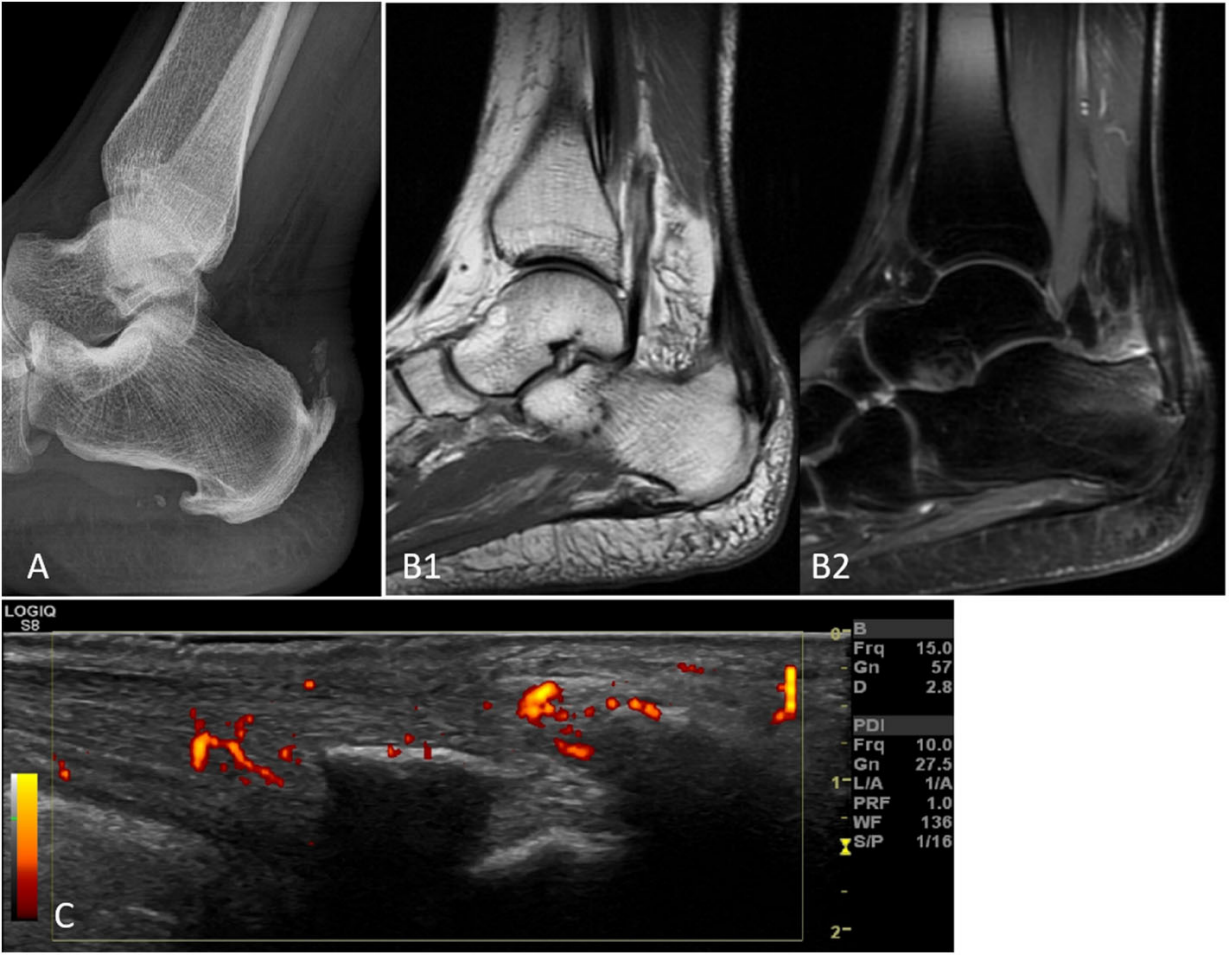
Achilles' tendon enthesitis of the same patients with peripheral SpA as assessed by XR (A), MRI with T1 sequences (B1), MRI with contrast-enhanced sequences (B2), and PD US (C). The various imaging techniques differently emphasize the pathological changes related to the disease, i.e., mainly bone changes with XR and soft tissue changes with MRI and US. Only MRI (B2) can document subcortical osteitis/bone marrow edema. Abbreviations: MRI, magnetic resonance imaging; SpA, spondyloarthritis; US, ultrasonography.
